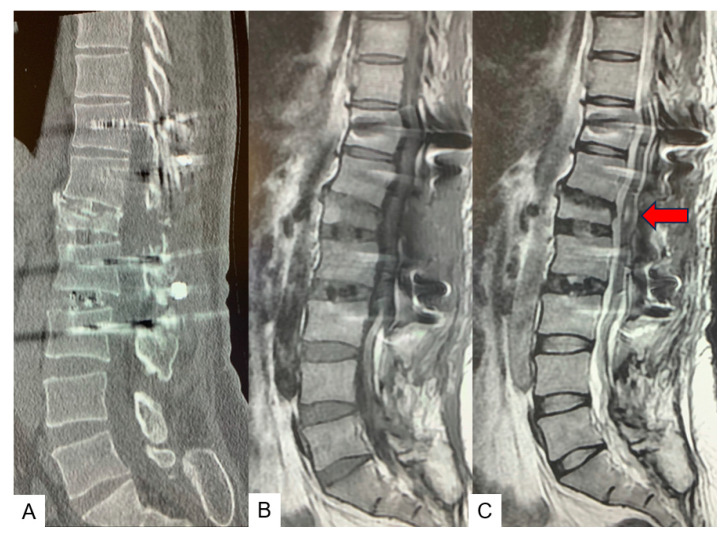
Follow-up images. (A) Mid sagittal reconstruction CT, (B) Mid sagittal T1-weighted MR imaging, (C) Mid sagittal T2-weighted MR imaging. There is as T2 high area in the spinal cord (red arrow).
